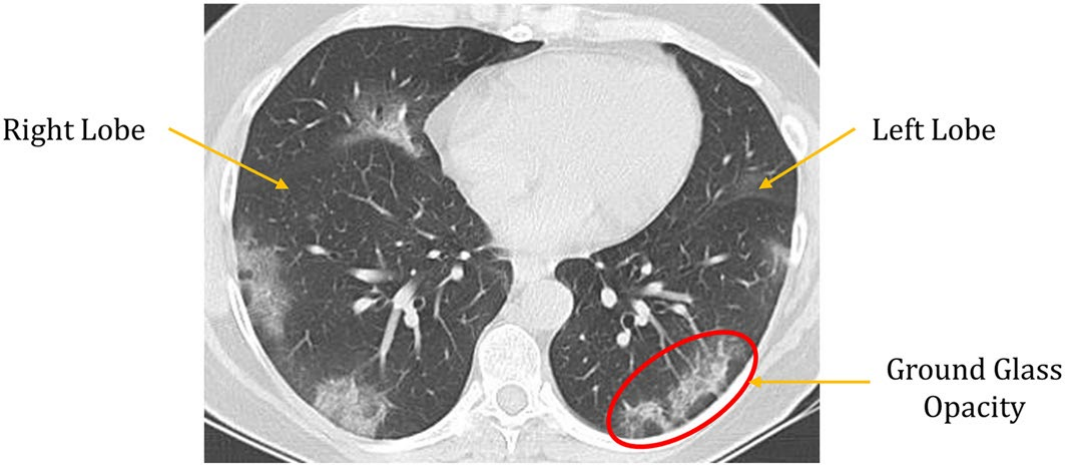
Example of a COVID infected Lung CT-scan image. The CT-scan image has been taken from the SARS-COV-2 dataset^15^. The ground glass opacity marked with the red circle in the image is the distinguishing feature of the COVID-19 infection.
